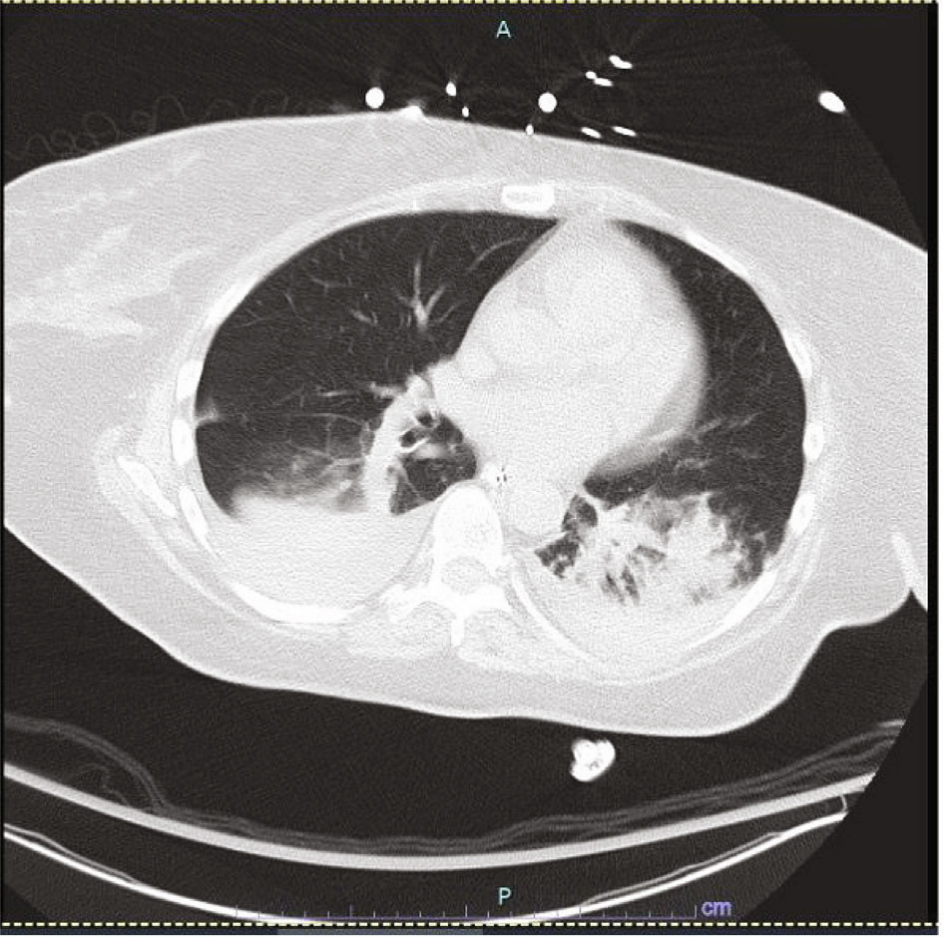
CT chest later in the course of hospitalization demonstrating bilateral basal opacities with pleural effusion.
